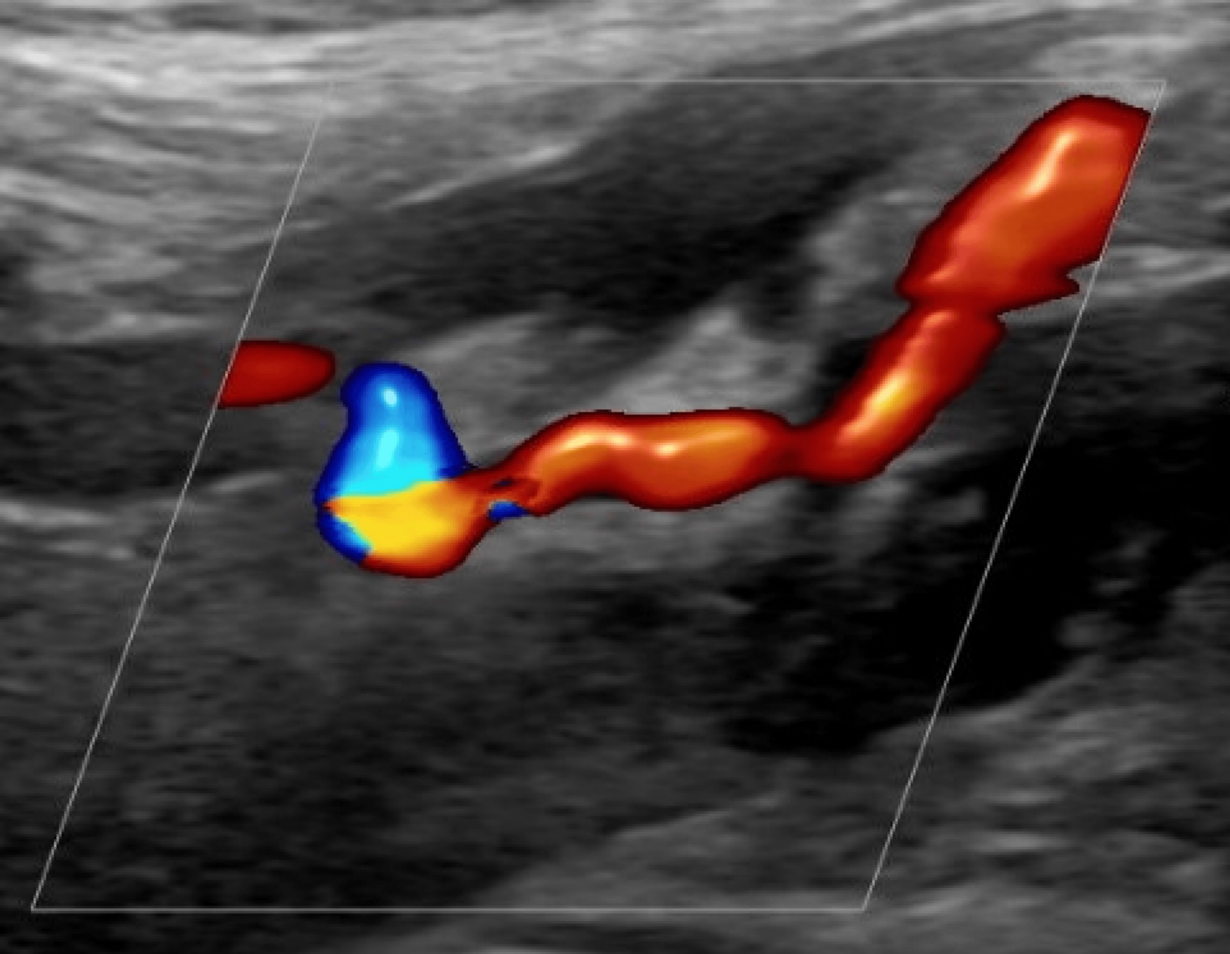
Left lower extremity arterial Doppler duplex ultrasound demonstrating severe atherosclerotic disease with occlusion of the mid and distal superficial femoral artery, popliteal artery, and dorsalis pedis artery
